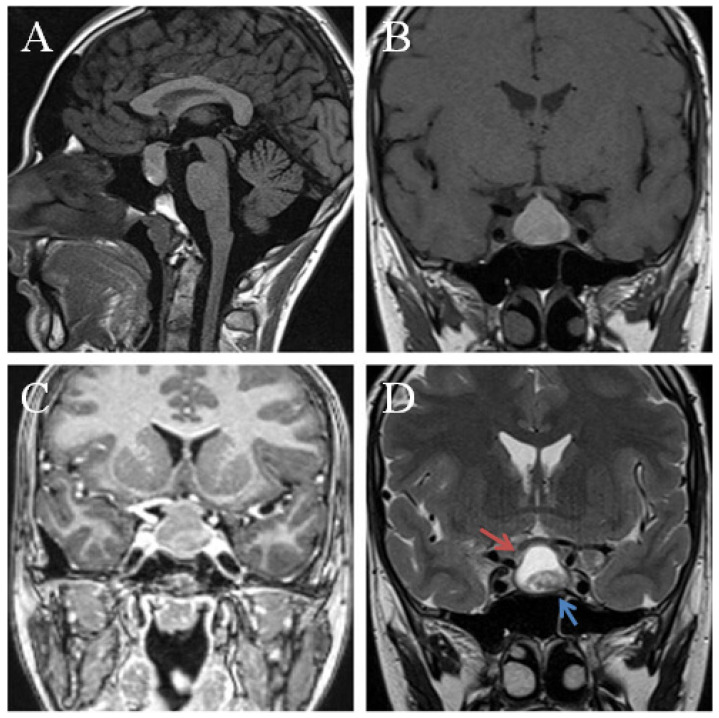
Preoperative MRI of a sellar lesion measuring 25 mm, and postoperatively diagnosed as xanthogranuloma of the sellar region. Sagital pre-contrast T1WI (A) demonstrates an isointense signal. The lesion was isointense on T1 precontrast sequences (B) with a corresponding mixed signal on T2 sequences (D) cystic component hyperintense (red arrow) and a hypointense solid component (blue arrow). Coronal postcontrast T1 (C) shows peripheral contrast enhancement. The tumor compressed the pituitary gland and the optic chiasma upward.
